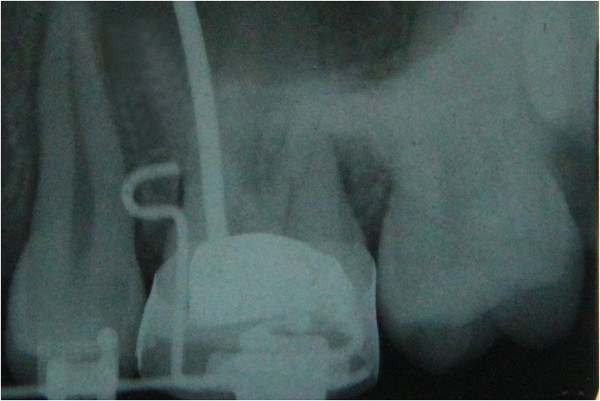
Intraoral periapical radiograph showing the position of the stent in the interradicular bone.
